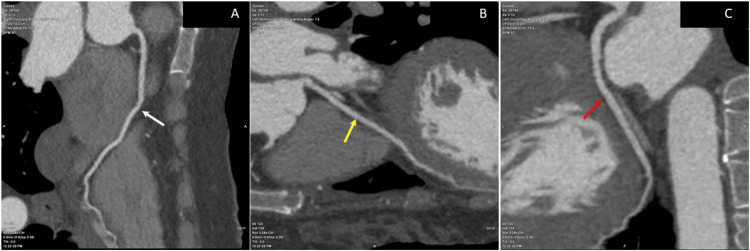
Processed images of a CT cardiac angiogram, showing normal coronary arteries outline and course, and no evidence of atherosclerotic coronary disease. (A) Right coronary artery. (B) Left anterior descending artery. (C) Left circumflex artery
